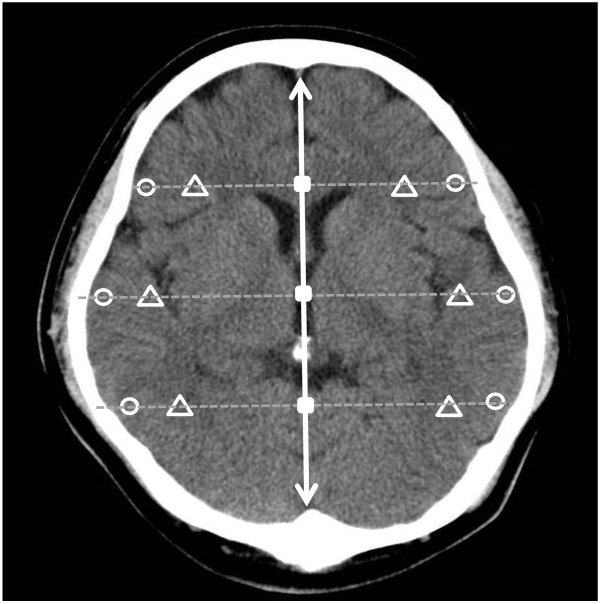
Measurement points of head computed tomography at the basal ganglial level. The indicated points were measured in Hounsfield units. Open circles indicate the positioning of the 10-mm^2^ regions of interest in the cerebral cortex. Open triangles indicate the positioning of the regions of interest in the white matter 1. Square symbols indicate the hallmarks placed to divide the distance from the frontal pole to occipital pole into four equal parts.
